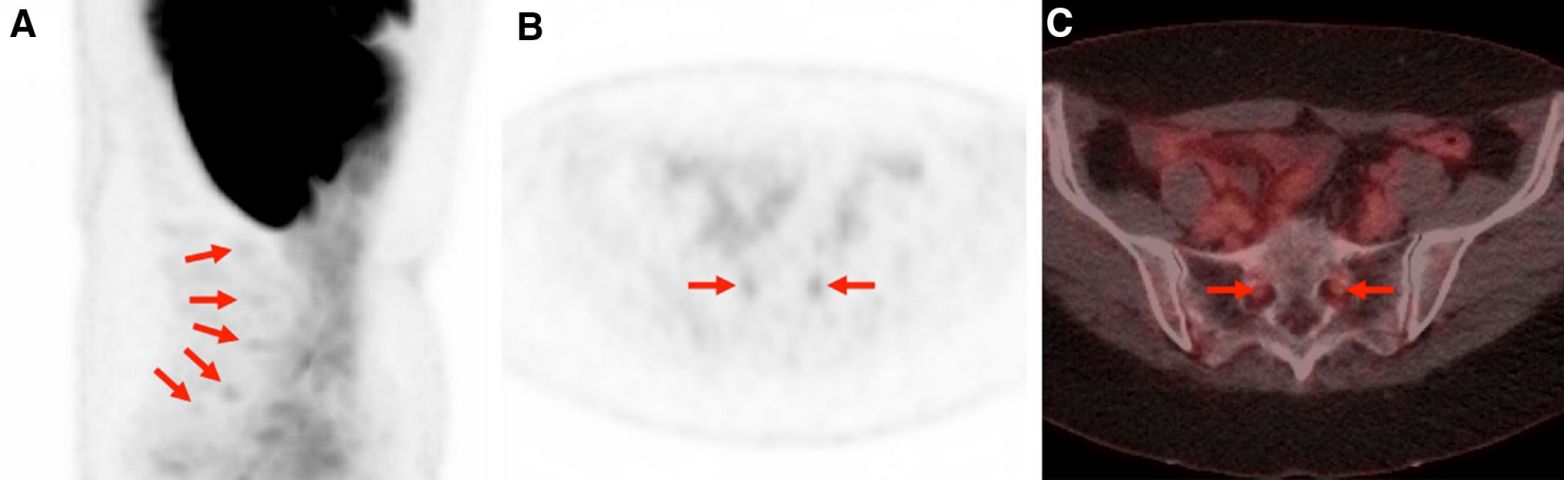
a Sagittal view of ^18^F-DCFPyL MIP image from a 58-year-old female patient with history of metastatic RCC and undergoing a staging examination. Multiple lumbar and sacral DRG show mild uptake (*red arrows*). b Axial ^18^F-DCFPyL PET and c axial ^18^F-DCFPyL PET/CT through the S1–2 level both demonstrate mild uptake of the S1 DRG (*red arrows*). Again, a portion of the normal physiologic biodistribution of the radiotracer is apparent including uptake in the patient’s remaining kidney, liver, and bowel
